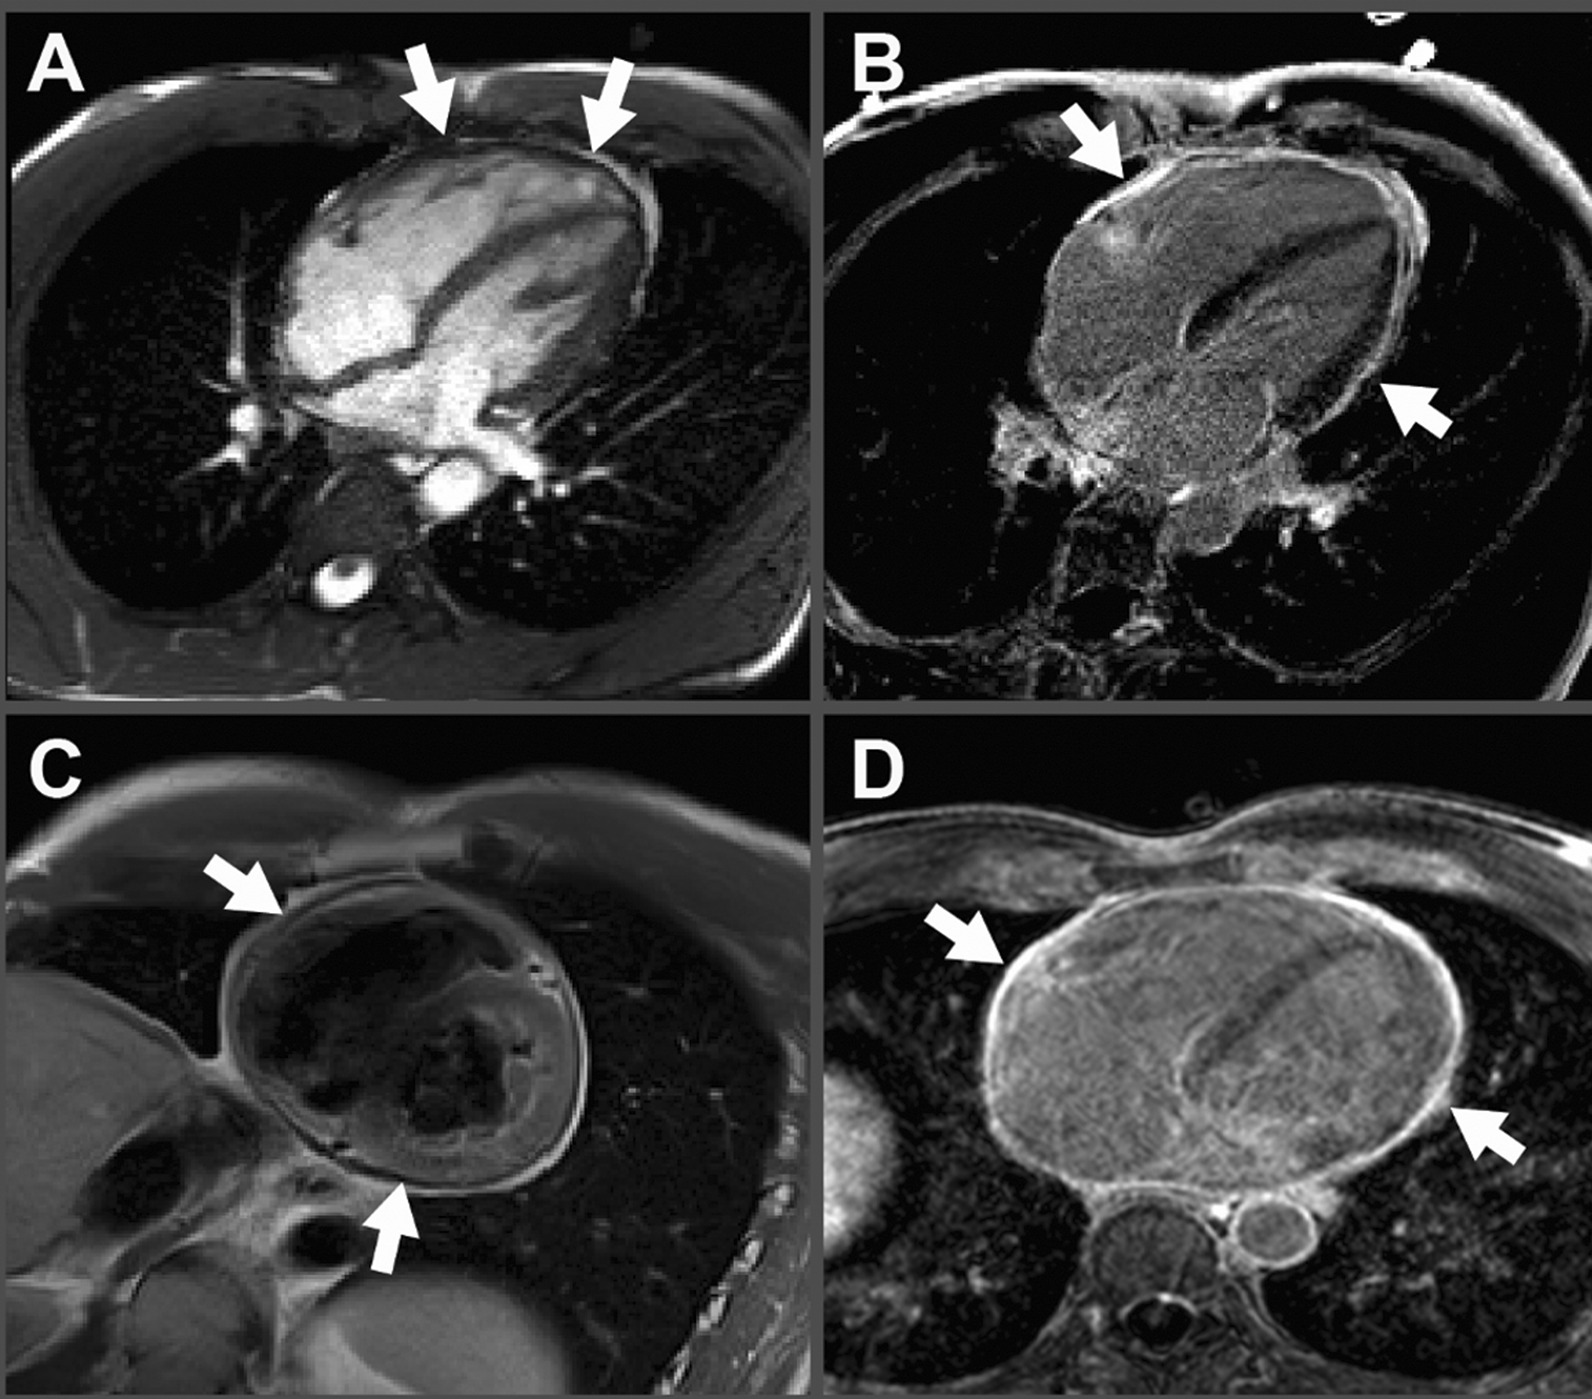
Cardiac magnetic resonance imaging. A Steady state free precession (“cine”) imaging demonstrates circumferential thickening of the pericardium (indicated with white arrows) and significant signal void consistent with calcification B Phase sensitive inversion recovery imaging approximately 10 min after administration of gadobenate dimeglumine demonstrates marked circumferential late gadolinium enhancement (indicated with white arrows) suggesting presence of inflamed and/or scar tissue C Double inversion recovery (“black blood”) imaging demonstrates marked circumferential thickening of the pericardium (indicated with white arrows) D Whole heart high resolution 3D inversion recovery imaging demonstrates pronounced late gadolinium enhancement of the entire pericardium (indicated with white arrows). No significant late enhancement of the myocardium was seen, suggesting lack of myocardial involvement or that any associated inflammatory process involving the myocardium has resolved without myocardial scar formation
